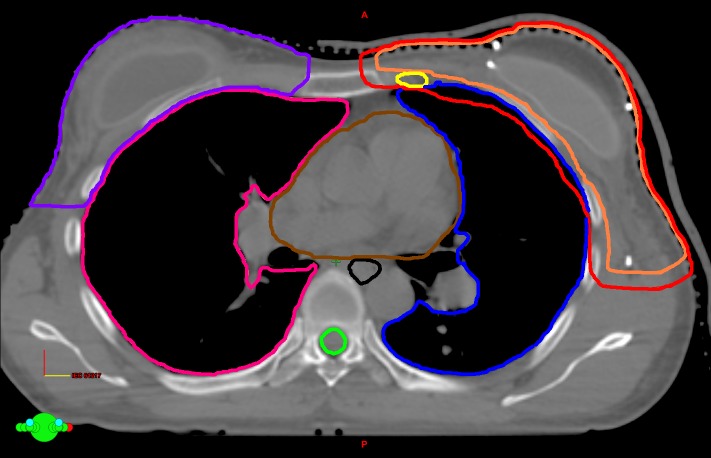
Target volumes and organ at risks (OARs) contoured on the axial slice of the planning computed tomography. Clinical target volume (orange), heart (brown), left lung (blue), internal mammary nodes (yellow), planning target volume (red) oesophagus (black), right breast (purple), right lung (pink), spinal cord (green)
